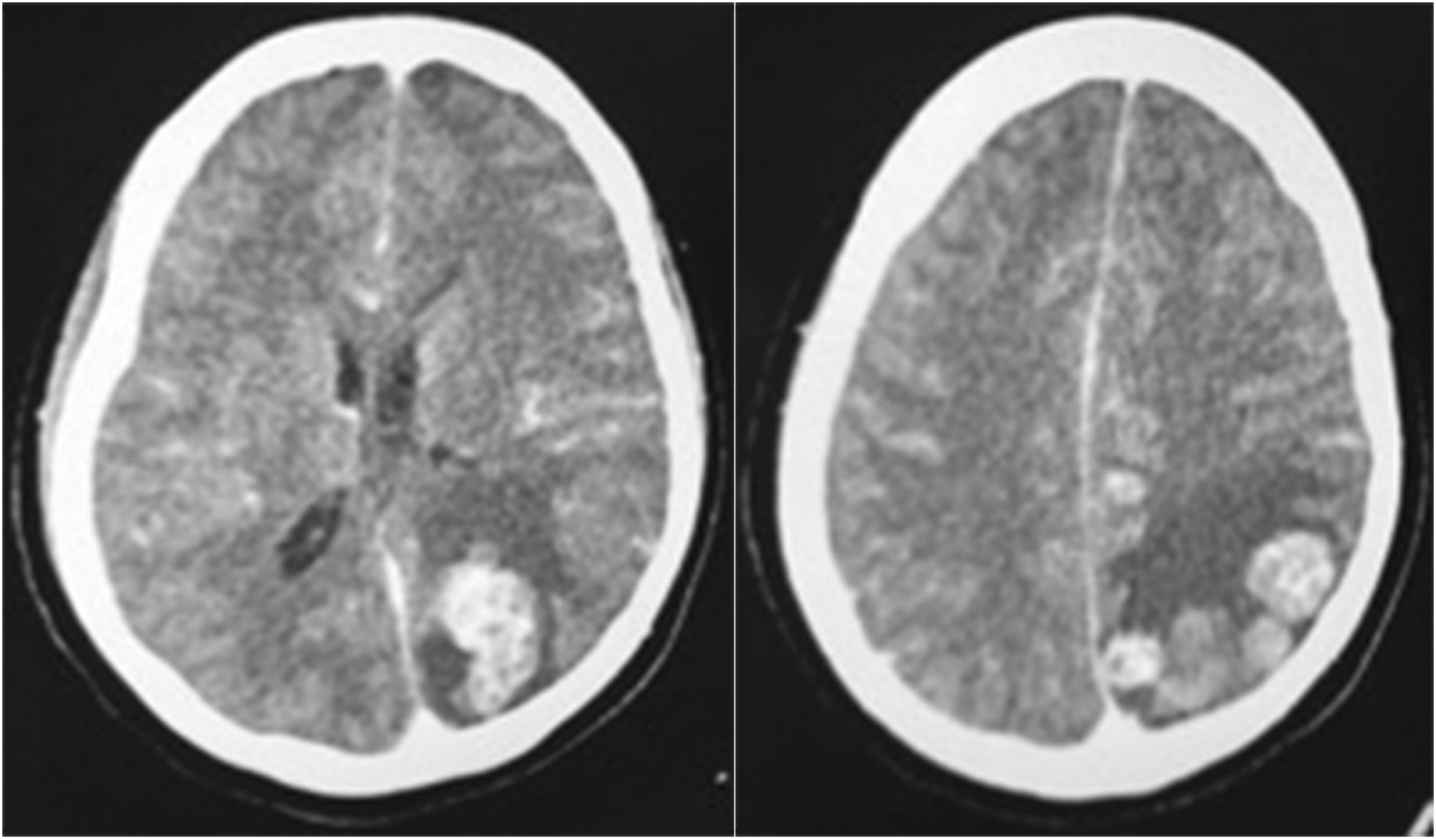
Case 1. Contrast‐enhanced head CT showed heterogeneously enhancing slightly hyperdense nodular masses of varying sizes with moderate perilesional edema in the left occipitoparietal region.
